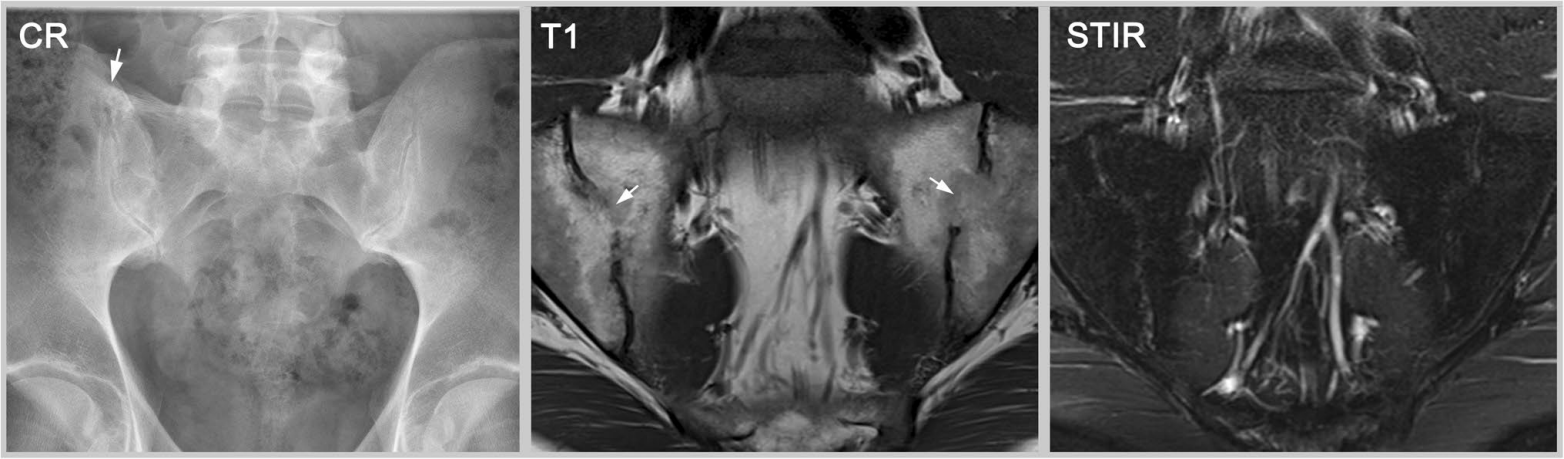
Established sacroiliitis not fulfilling the ASAS definition of a positive MRI. Despite radiography that only shows some capsule calcification (arrow), MRI-T1 clearly displays bilateral ankylosis in the midpart of the joint. The ankylosis is not amenable to detection by radiography because this part of the joint is not in parallel orientation to the X-ray beam. MRI-STIR shows no osteitis. This patient with established axSpA fulfills neither the radiographic standard (mNYC is negative) nor the ASAS definition for a positive MRI (no bone marrow edema) and represents another example of ‘false-negative’ imaging if the ASAS definitions are used for clinical diagnosis
